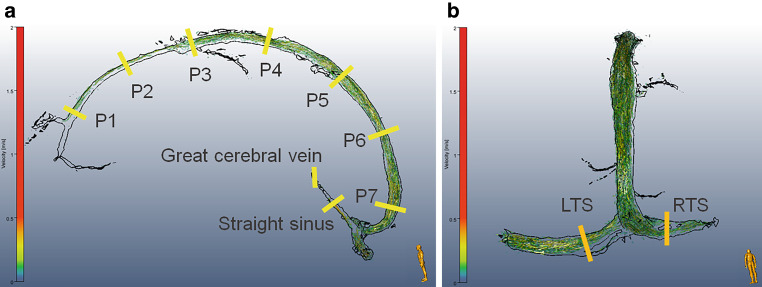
Visualization of intracranial venous flow using 4D Flow MRI in birds’ view perspective. The systematic analysis includes seven equidistant cross-sections within the superior sagittal sinus (P1–P7), and one cross-section within the great cerebral vein (vena cerebri magna, VCM), straight sinus (a sagittal view), left (LTS) and right (RTS) proximal transverse sinus (b coronal view). Color coding represents the velocity [m/s] of flow trajectories
